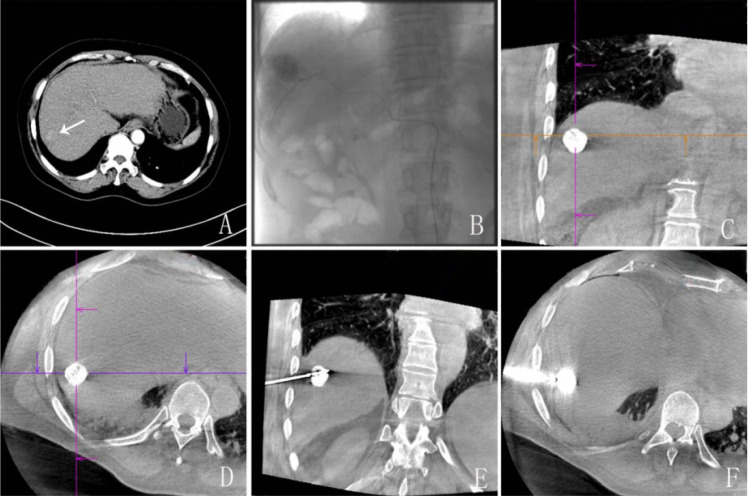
A 61-year-old woman with a 20-year history of hepatitis B and cirrhosis. (A) CT showed a 3.3-cm diameter lesion near the diaphragmatic dome, and biopsy showed hepatocellular carcinoma. (B) Iodized oil emulsion was used to embolize the blood supply artery of the tumor. (C) CT examination after transcatheter arterial chemoembolization (TACE) showed good Lipiodol deposition. (D–F) Microwave ablation (MWA) was performed under the guidance of DynaCT 1 week after TACE. Power was set to 60 W and ablated two target lesions for a total of 12 min.
